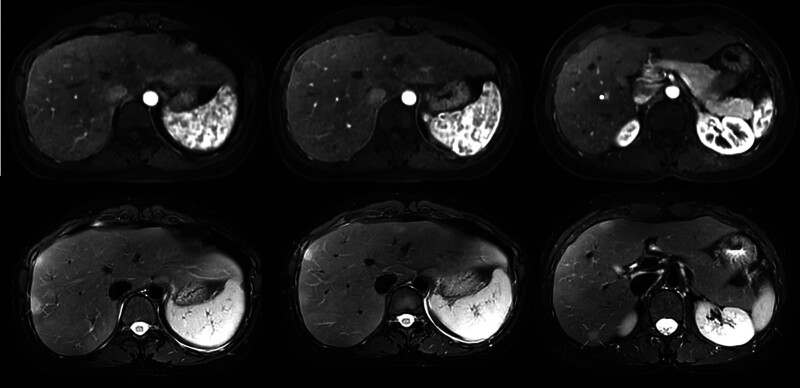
Liver 4Dflow magnetic resonance imaging in April 20, 2024: arterial enhancement phase on T1-weighted image and nonarterial enhancement phase on T2-weighted image.
